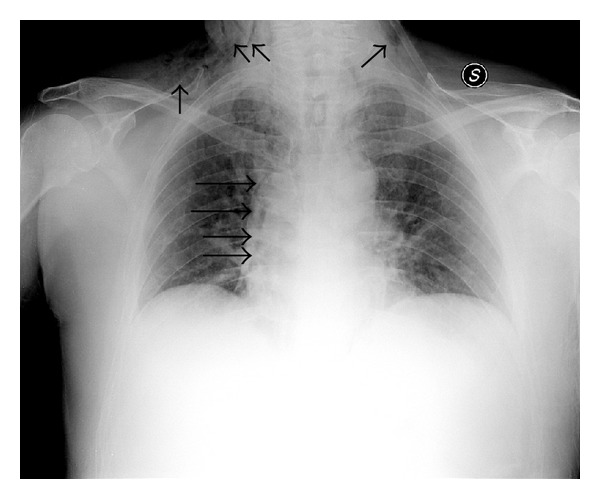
Chest X-ray showed subcutaneous emphysema, with gas tracking into the neck area bilaterally and a paracardiac gas stripe of the right (black arrows).
